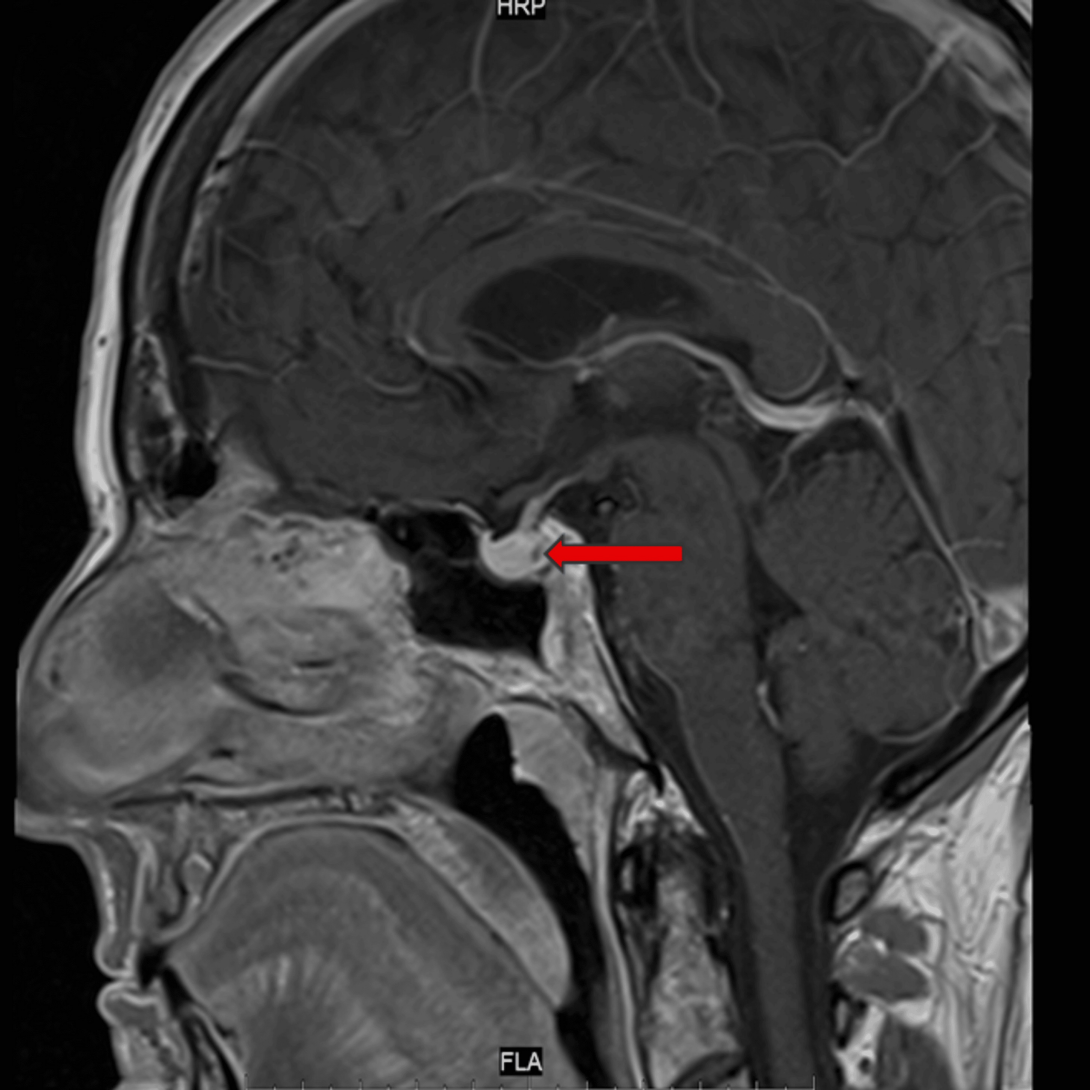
Sagittal post-contrast pituitary MRI showing a 2 mm hypoenhancing microadenoma (MA) in the anterior lobe
